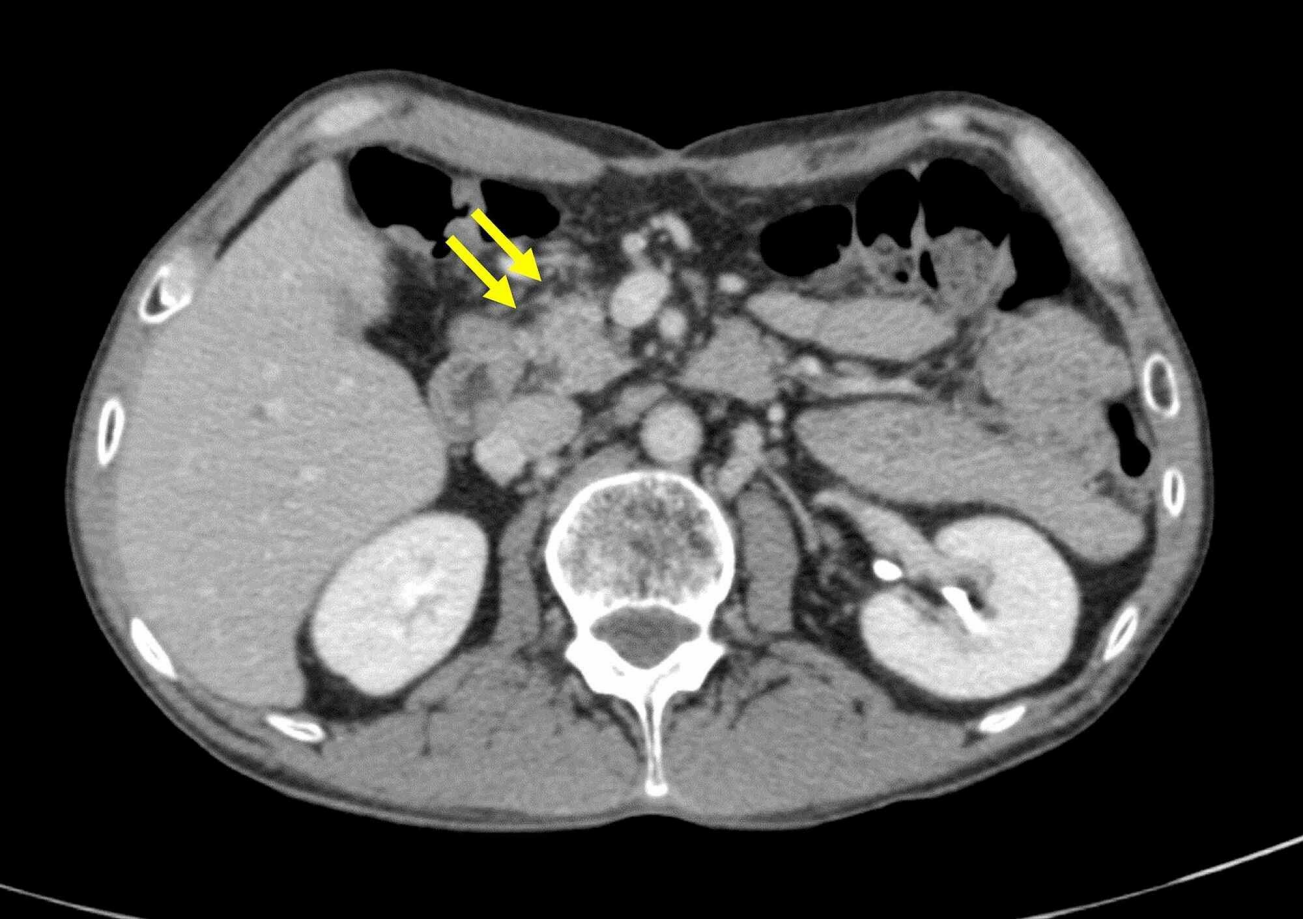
Computed tomographic scans of the abdomen with contrast.
